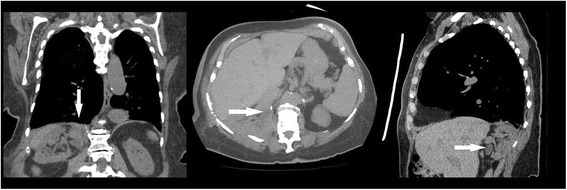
Post-surgical computed tomography (CT) demonstrating repair of diaphragmatic hernia and segmental resection of ureter. Coronal, Axial, Sagittal views with white arrow showing resolution of hydroureteronephrosis and repair of diaphragmatic hernia. Note the changes in the position of the right kidney from nephroxy and no redundant ureter is evident
